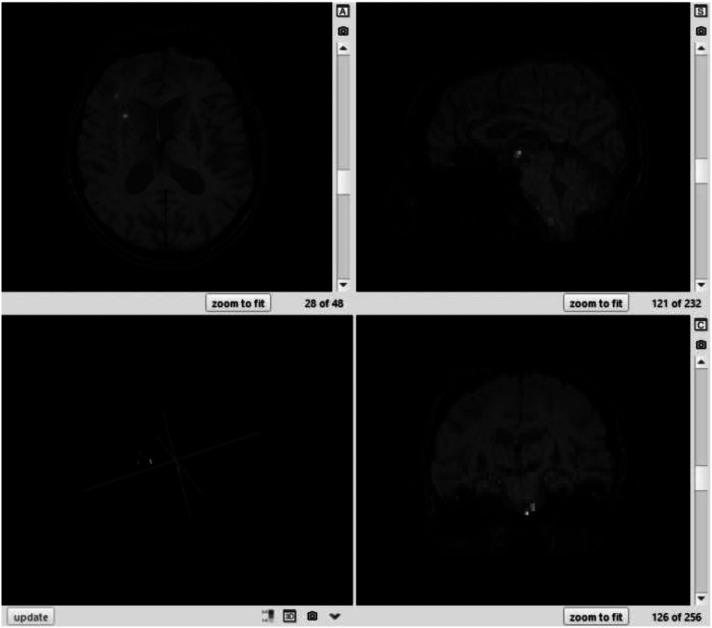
Representative multiplanar ITK-SNAP view demonstrating manual placement of color-coded anatomical landmarks on axial magnetic resonance imaging with corresponding sagittal, coronal, and 3D spatial coordinate visualization.
